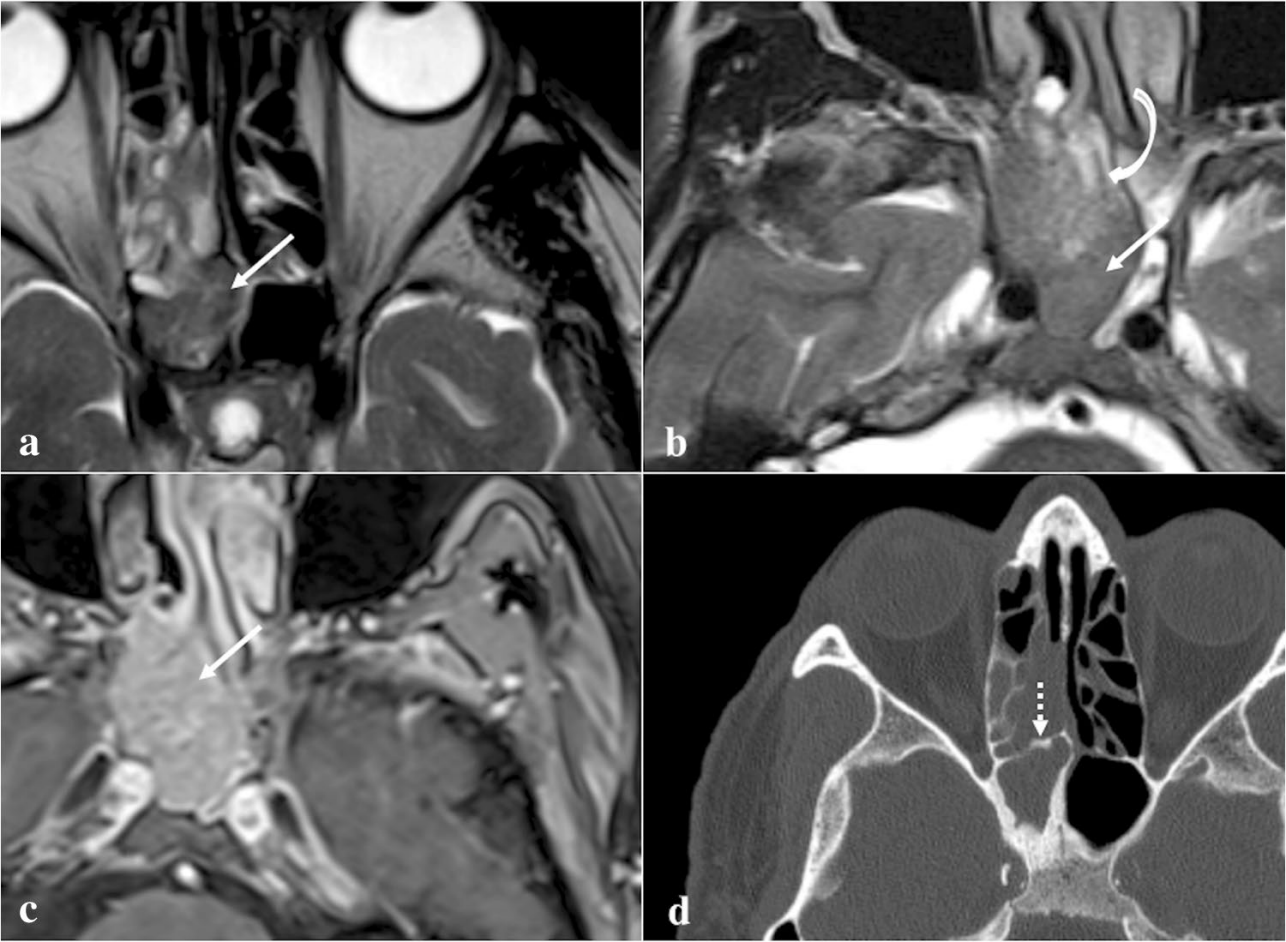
Inverted papilloma of the right sphenoid sinus in a 71-year-old male patient. MRI shows a solid expansive lesion in the right nasal fossa in correspondence to the spheno-ethmoidal recess (white arrows). That lesion has similar SI to the grey matter on axial T2W images (a and b) with focal “cerebroid” appearance (b, white curved arrow) and moderate enhancement on T1W fat-saturated CE image (c). Axial bone reconstruction algorithm CT reveals a focal plaque-like hyperostosis in the anterior wall of the right sphenoid sinus (d, white dotted arrow), as the likely site of tumour origin
